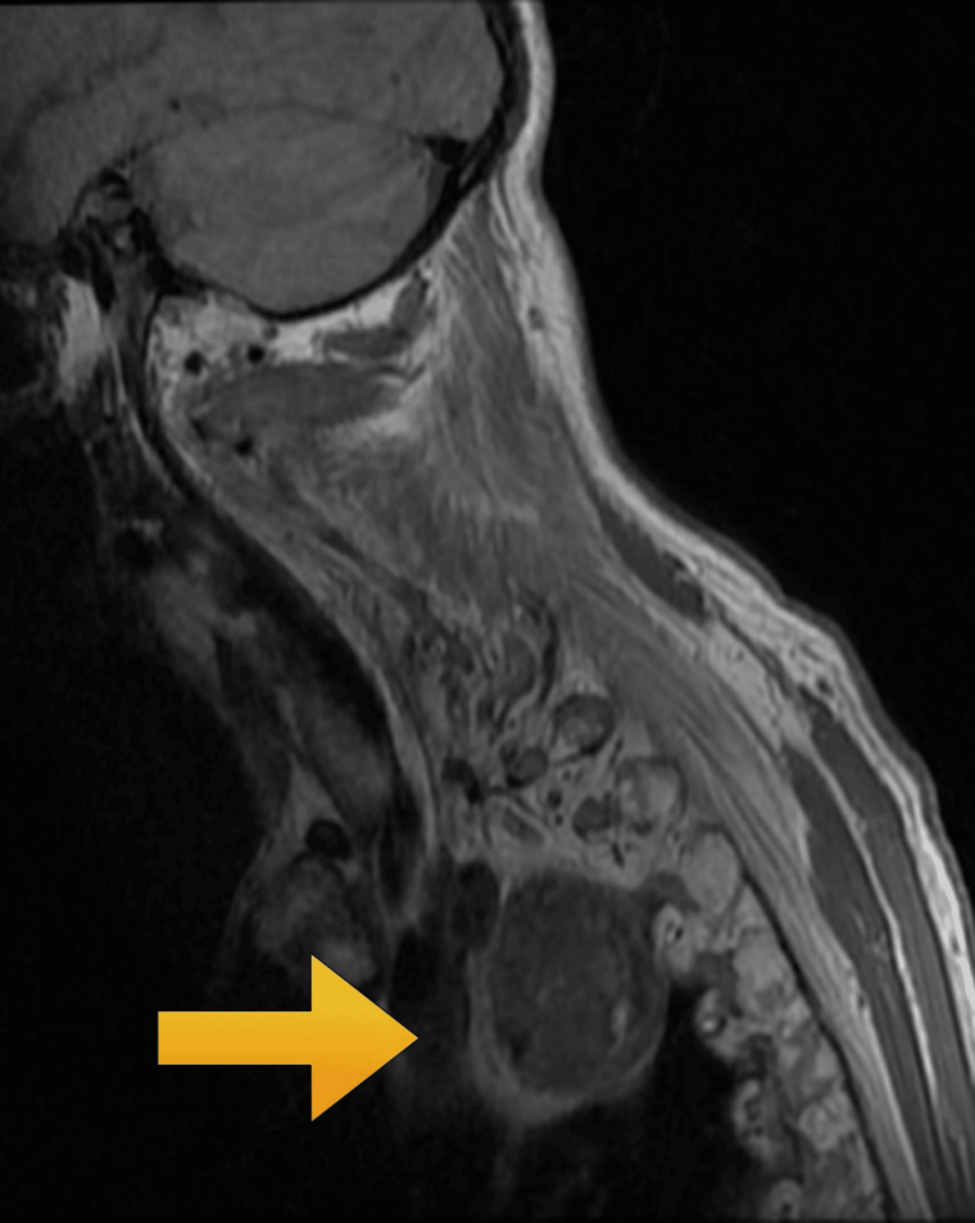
Sagittal T2-weighted MRI of the cervicothoracic junction demonstrating diffuse ossification of the anterior longitudinal ligament and loss of normal cervical lordosis, consistent with ankylosing spondylitis. Additionally, there is a well-defined, T2 hyperintense mass lesion in the posterior mediastinum, located anterior to the upper thoracic vertebrae and extending from approximately C7 to T3 vertebral levels. The lesion appears to displace adjacent soft tissue structures without frank invasion The yellow arrow shows the posterior mediastinal mass in the MRI. MRI: magnetic resonance imaging; C7: seventh cervical vertebra; T3: third thoracic vertebra
